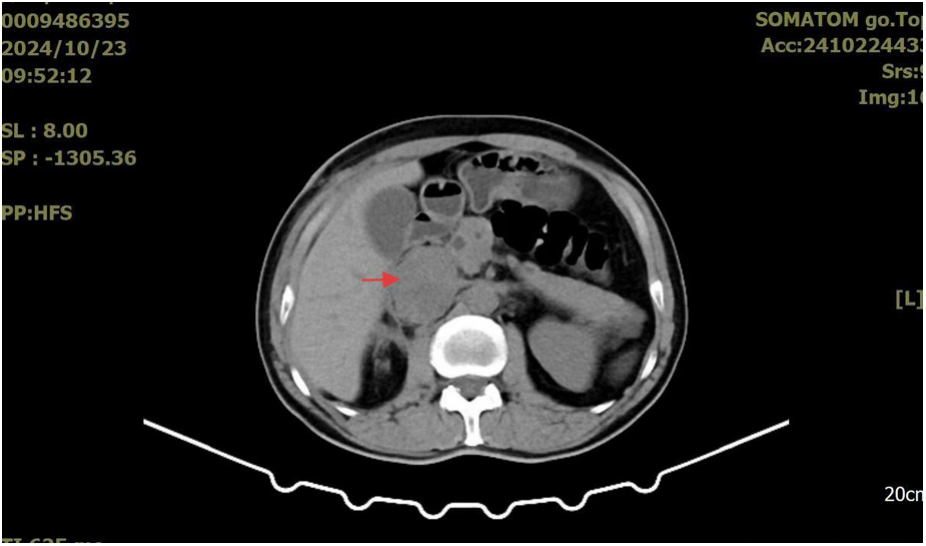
Postoperative CT scan reveals mass around inferior vena cava.
